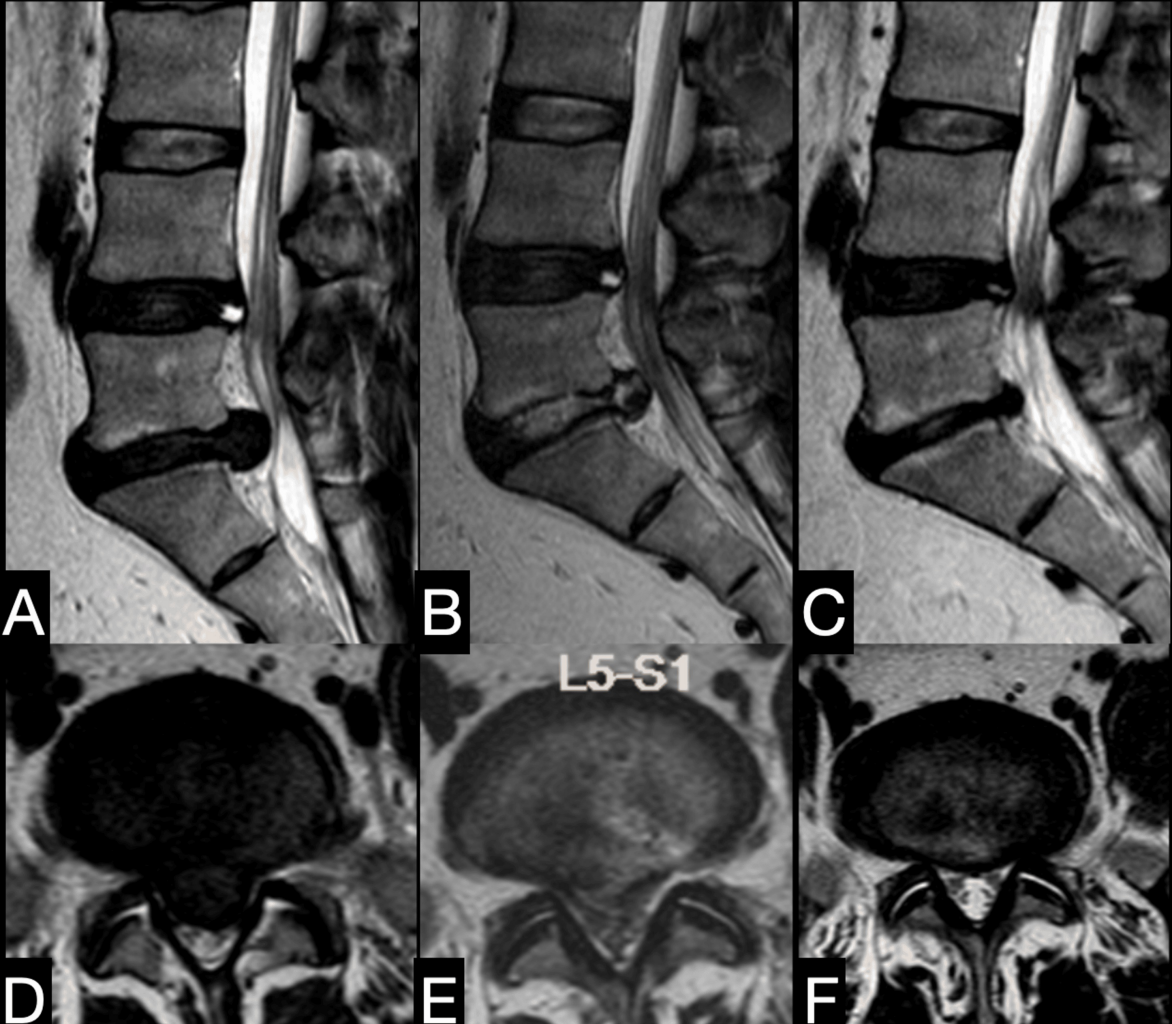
Large central disc operated by transiliac transforaminal endoscopic lumbar discectomy with adequate decompression demonstrable on postoperative MRI. (A, D) MRI of a 49-year-old male with a high iliac crest showing L5-S1 big disc prolapse, Michigan State University Classification 3AB, central disc prolapse with non-symptomatic L4-5 annular tear. The patient was operated on using transiliac transforaminal endoscopic discectomy. (B, E) Immediate postoperative MRI shows adequate decompression. The patient had significant relief of symptoms. (C, F) Nine-year follow-up MRI with maintained radiological and excellent clinical outcome at the L5-S1 and static L4-5 annular tear.
